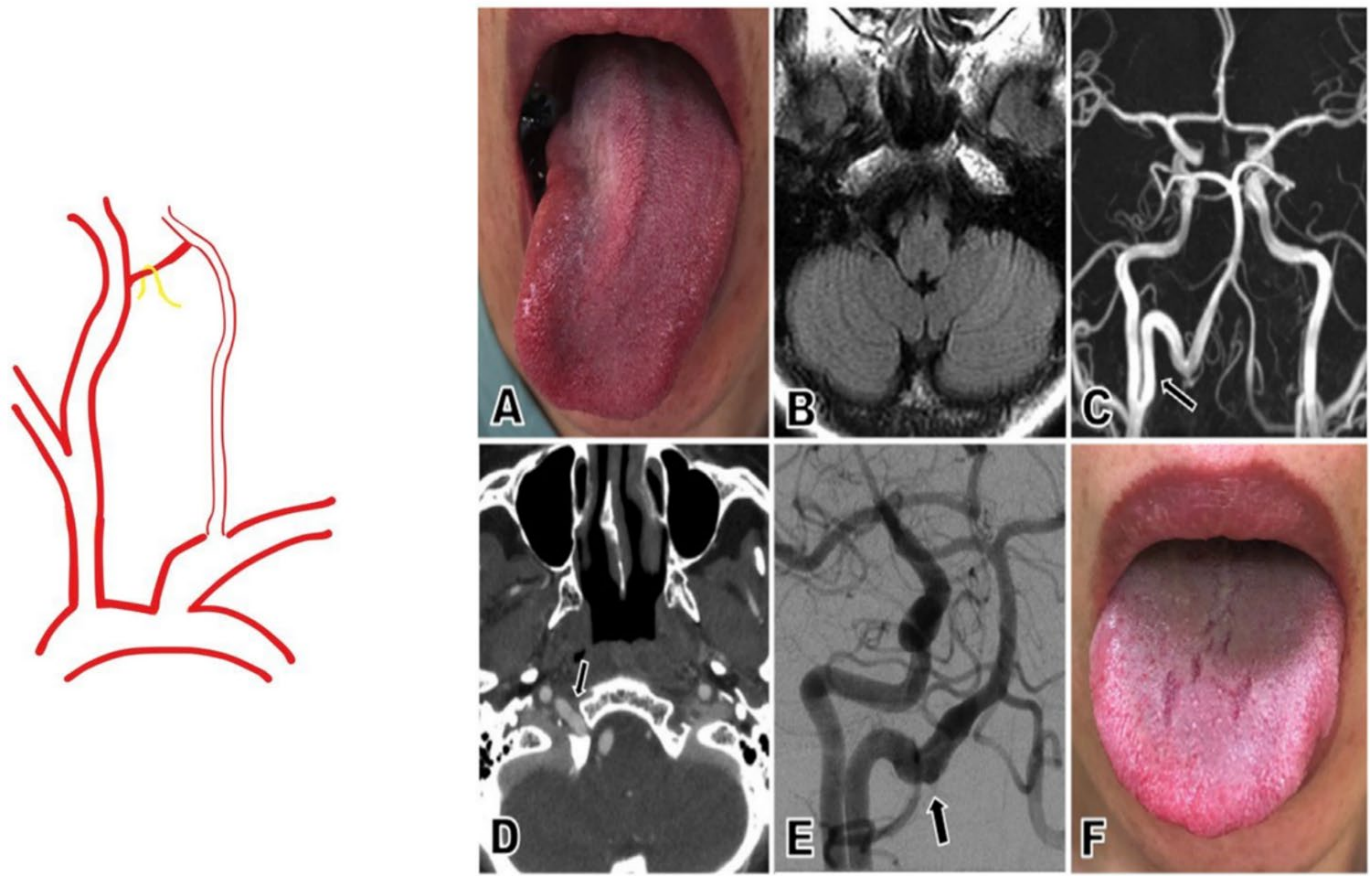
Category V PPHA: PPHA with cranial nerve compression. (A) Upon admission, neurological examination revealed rightward deviation of the patient’s tongue. (B) FLAIR imaging showed no evidence of pathology in the medulla oblongata. (C) Magnetic resonance angiography identified an unusual vessel arising from the right internal carotid artery (arrow). (D) CT angiography demonstrated this artery entering the skull via the right hypoglossal canal (arrow). (E) Digital subtraction angiography revealed an elongated persistent primitive hypoglossal artery with an irregular lumen within the hypoglossal canal (arrow). (F) The patient's right hypoglossal nerve palsy showed improvement one year after symptom onset [18]. Reproduced with permission from Hikichi et al., J Stroke Cerebrovasc Dis. 2020;29(2):104,459. © 2020 Elsevier. All rights reserved
